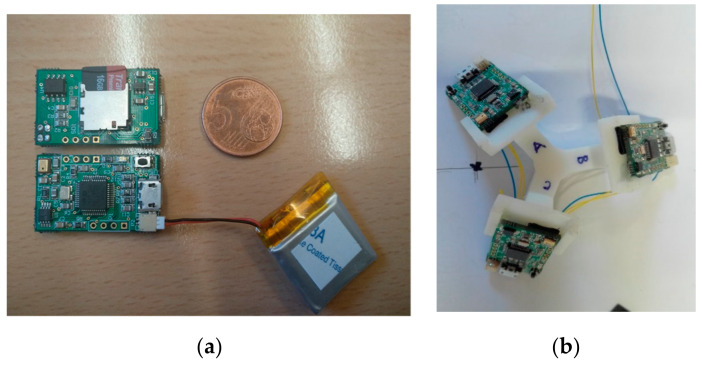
General aspect of the reception module: *(*a) a single ultrasonic receiver; (b) a multiple receiver prototype.
